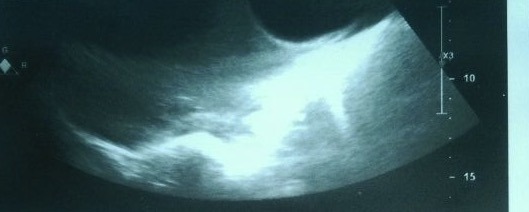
Right pleural sonography shows ruptured hydatid cysts
